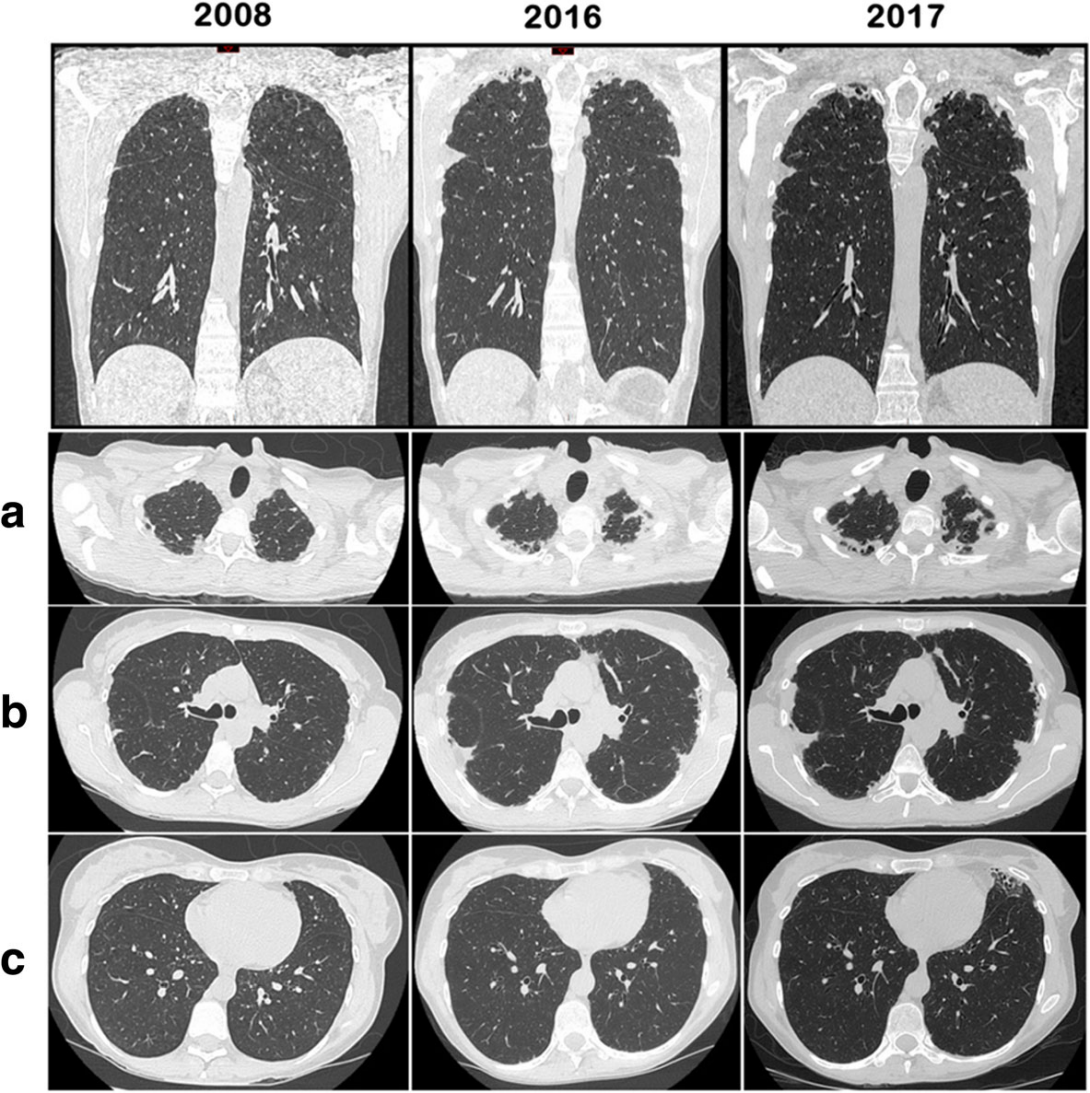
Evolution of thoracic imaging by CT-scan over time. Axial images centered on a 2nd thoracic vertebra, b 5th thoracic vertebra and c 8th thoracic vertebra. Pleural and pulmonary injuries are characterized by fibrotic foci of the upper lobes, without any other signs of SSc-related ILD. An apico-basal gradient of lesions was found. A slow extention of the fibrosis was seen in 2016 and 2017
